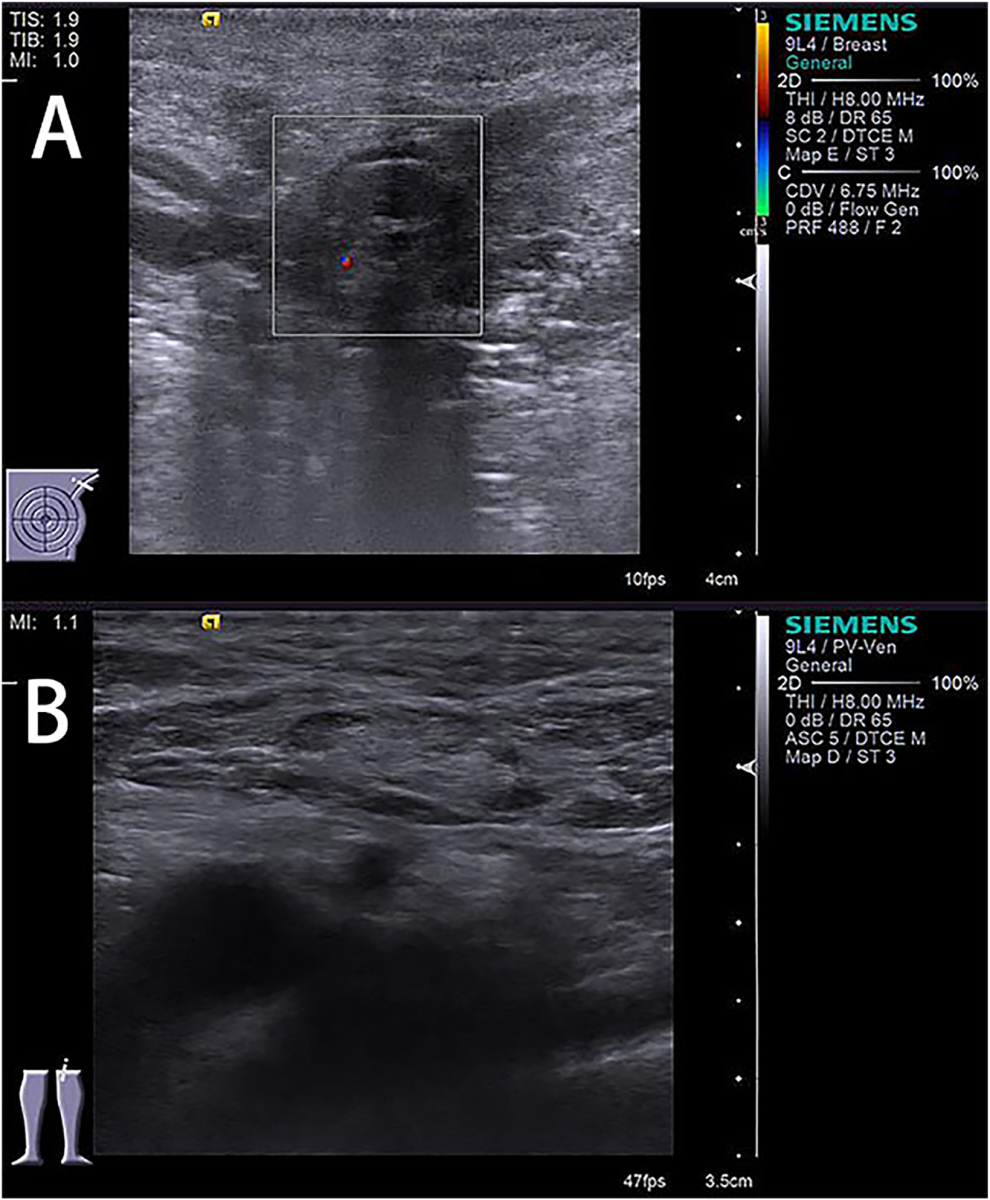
Color Doppler ultrasound images of superficial lymph nodes. (A) Shows enlarged bilateral axillary lymph nodes; (B) displays enlarged bilateral inguinal lymph nodes.
